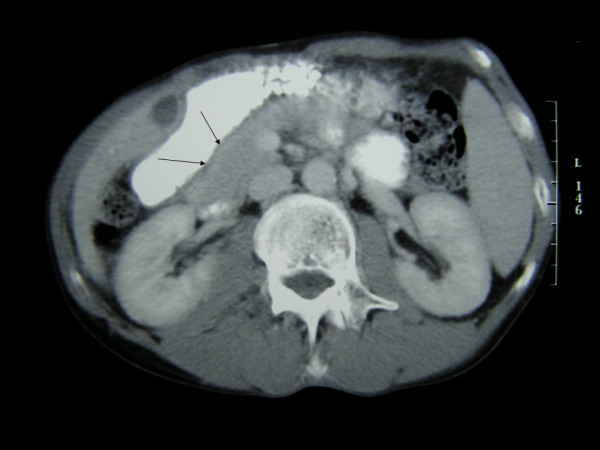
Computed tomography of the abdomen depicting a mass (arrows) in the duodenum.
